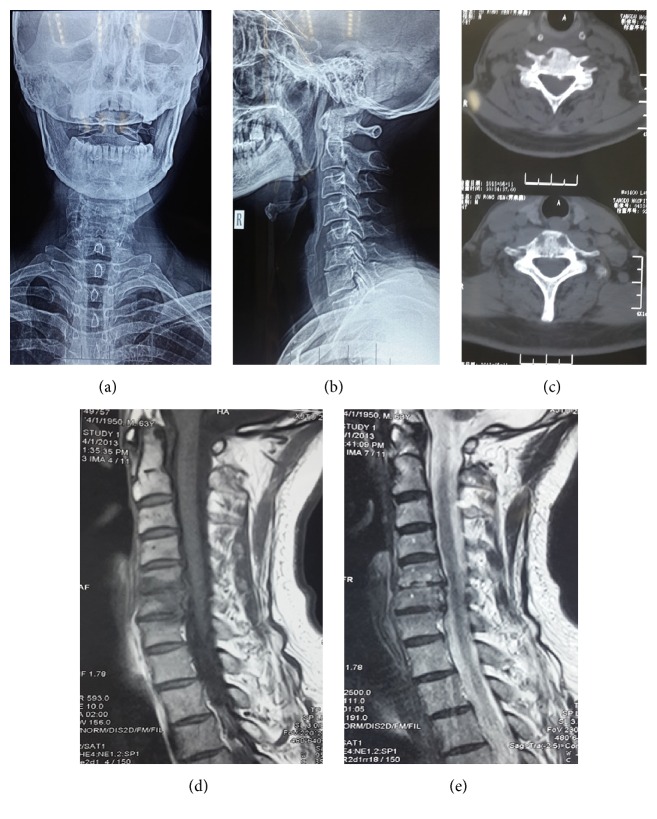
A 65-year-old male sustained fever and testicular pain for 1 week. After receiving treatment at the urinary surgery department for 1 week, the symptoms were relieved, but cervical pain emerged after another week. The anteroposterior and lateral plain of the cervical spine revealed narrow intervertebral spaces of C5-6 and C6-7. The CT scan revealed destruction of the cortical and cancellous bone. The edges of the centrums were sclerotic, but sequestrum was not found. T1 and T2 MRI revealed that the intensity of the C5-6 was abnormal and that the intervertebral space was narrow. The brucella serum agglutination test result exceeded 1 : 160. Brucella blood culture was negative. The patient was diagnosed with brucella infection.
